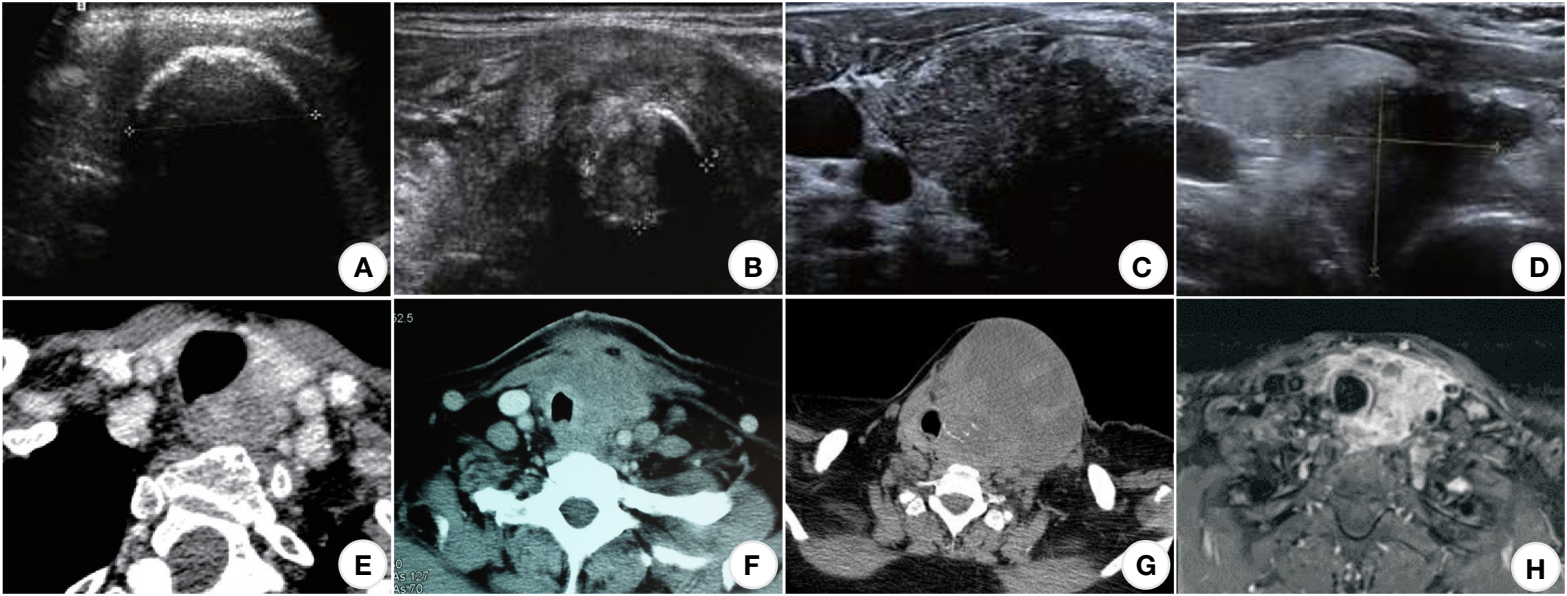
US, CT, and MRI images of SCCT. (A) (US) Eggshell-shaped calcification on the surface of the nodule; internal echoes are unavailable (33); (B) (US) Ring-shaped calcifications within the nodule (33); (C) (US) Punctate calcification within the nodule (33); (D) (US) Hypoechoic nodule with unclear borders, breaking through the thyroid peritoneum and invading the surrounding tissue (33); (E) (CT) Uneven moderate enhancement of the nodules, the esophagus and trachea were compressed and displaced, and the boundary was unclear; (F) (CT) The mass compresses and infiltrates the trachea and surrounding soft tissues (36); (G) (CT) Partial necrosis of the mass in the left lobe of the thyroid infiltrating adjacent skin and trachea (31); (H) (MRI) Uneven enhancement of the left lobe of the thyroid, with unclear boundaries with the esophagus, trachea, and left common carotid artery (37).
